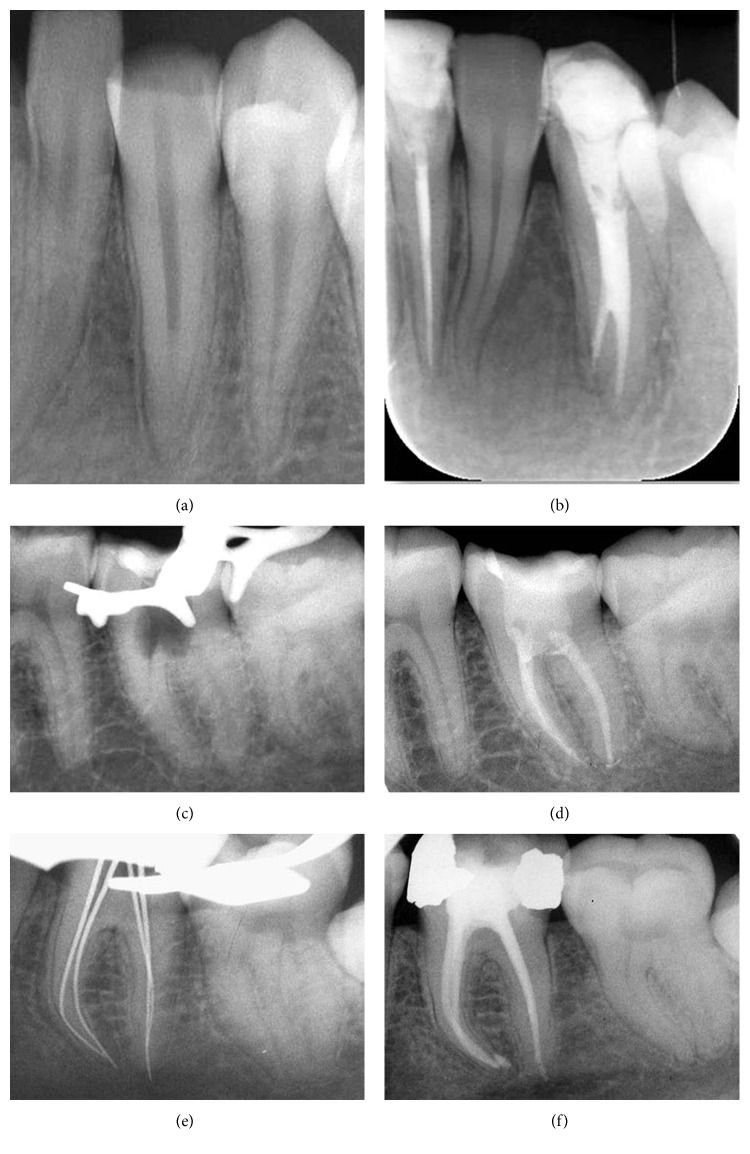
Radiographic views of root canal fillings. (a) Preoperative view of tooth 33; (b) obturation with SystemB/Obtura II; (c) accidental perforation on tooth 37; (d) sealing of the perforation with resin-modified glass ionomer cement and canal filling with SystemB/ObturaII; (e) retroalveolar view of tooth 36; (f) obturation with Thermafil.
